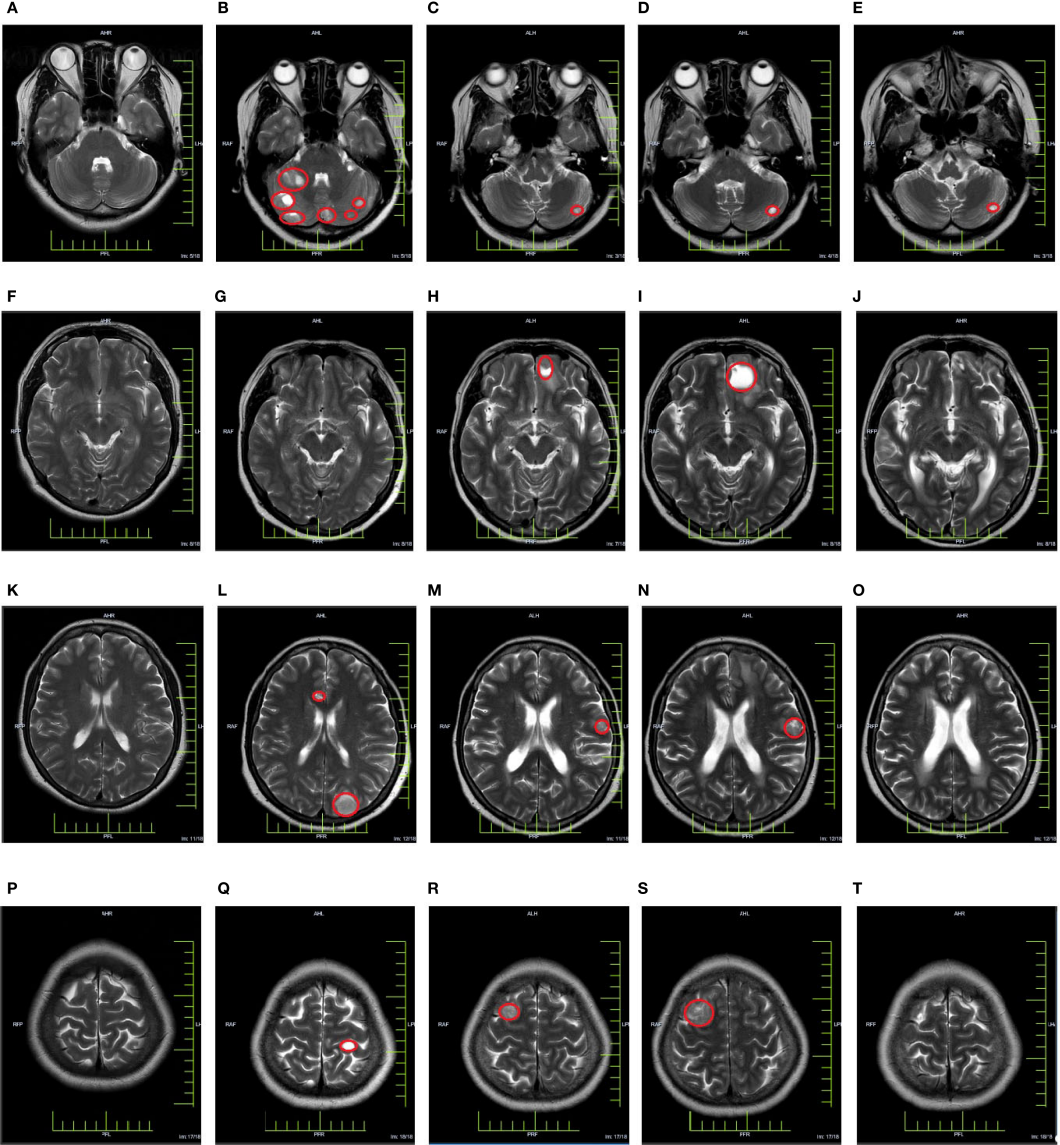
Cranial MRI evaluation map [(A, F, K, P) In March 2021, a baseline cranial MRI evaluation was performed, revealing no apparent abnormalities in the cranium. (B, G, L, Q) In March 2022, a follow-up cranial MRI examination was conducted, revealing the presence of multiple metastatic lesions. (C, H, M, R) In March 2023, a follow-up cranial MRI examination was conducted, revealing the presence of newly detected lesions in the cranium (D, I, N, S) In October 2023, a follow-up cranial MRI examination was performed, revealing an increasing trend in the size of the lesion. (E, J, O, T) In December 2023, a follow-up cranial MRI examination was conducted, revealing a significant reduction or disappearance of metastatic lesions].
